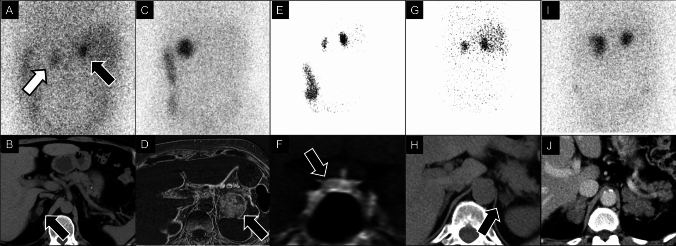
Posterior to anterior view of ^131^I-adosterol scintigraphy and related imaging findings under various conditions. (A) and (B): Subclinical Cushing syndrome in a 50-year-old female. Adrenocortical scintigraphy showing moderate radiotracer uptake in the right-adrenal mass (A: black arrow) with mild contralateral adrenal suppression (A: white arrow), suggesting subclinical Cushing’s syndrome. Non-contrast computed tomography (CT) showing a hypo-attenuating mass on right-adrenal gland consistent with adenoma (B: black arrow). (C) and (D): Cushing syndrome in a 60-year-old female. Adrenocortical scintigraphy showing marked radiotracer uptake in the adenoma with obvious contralateral suppression, indicative of Cushing syndrome (C). Chemical-shift imaging subtraction image highlights the fat content in the adenoma (D: black arrow). (E) and (F): ACTH-dependent Cushing’s disease in a 42-year-old female. Bilateral adrenal uptake is detected by adrenocortical scintigraphy (E), and pituitary magnetic resonance imaging reveals a hypovascular adenoma on delayed contrast-enhanced imaging (F: black arrow). G and H: Bilateral micronodular adrenocortical disease in a 67-year-old female. Although initially suspected as a unilateral functional adrenal adenoma by CT (H), adrenocortical scintigraphy showed symmetrical high uptake bilaterally, leading to the diagnosis of bilateral micronodular adrenocortical disease, preventing surgery (G). I and J: Bilateral macronodular adrenocortical disease in a 71-year-old male. Adrenocortical scintigraphy showing high uptake bilaterally (I) with multiple nodules on contrast-enhanced CT (J), leading to the diagnosis of bilateral macronodular adrenocortical disease.
